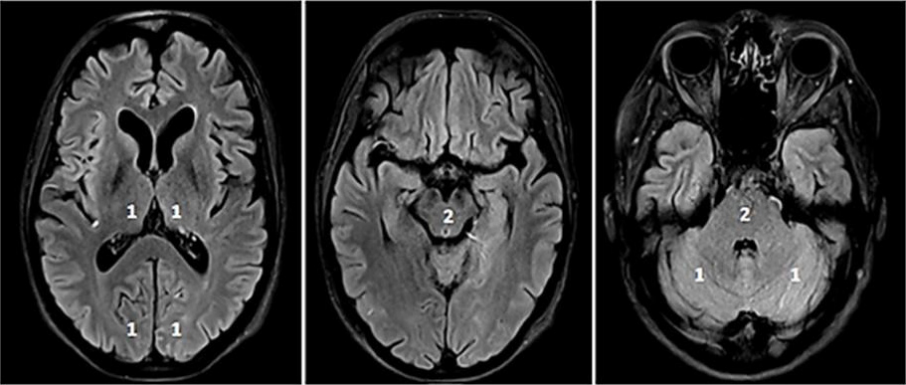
pc-ASPECTS (posterior circulation ASPECTS). The pc-ASPECTS is a
